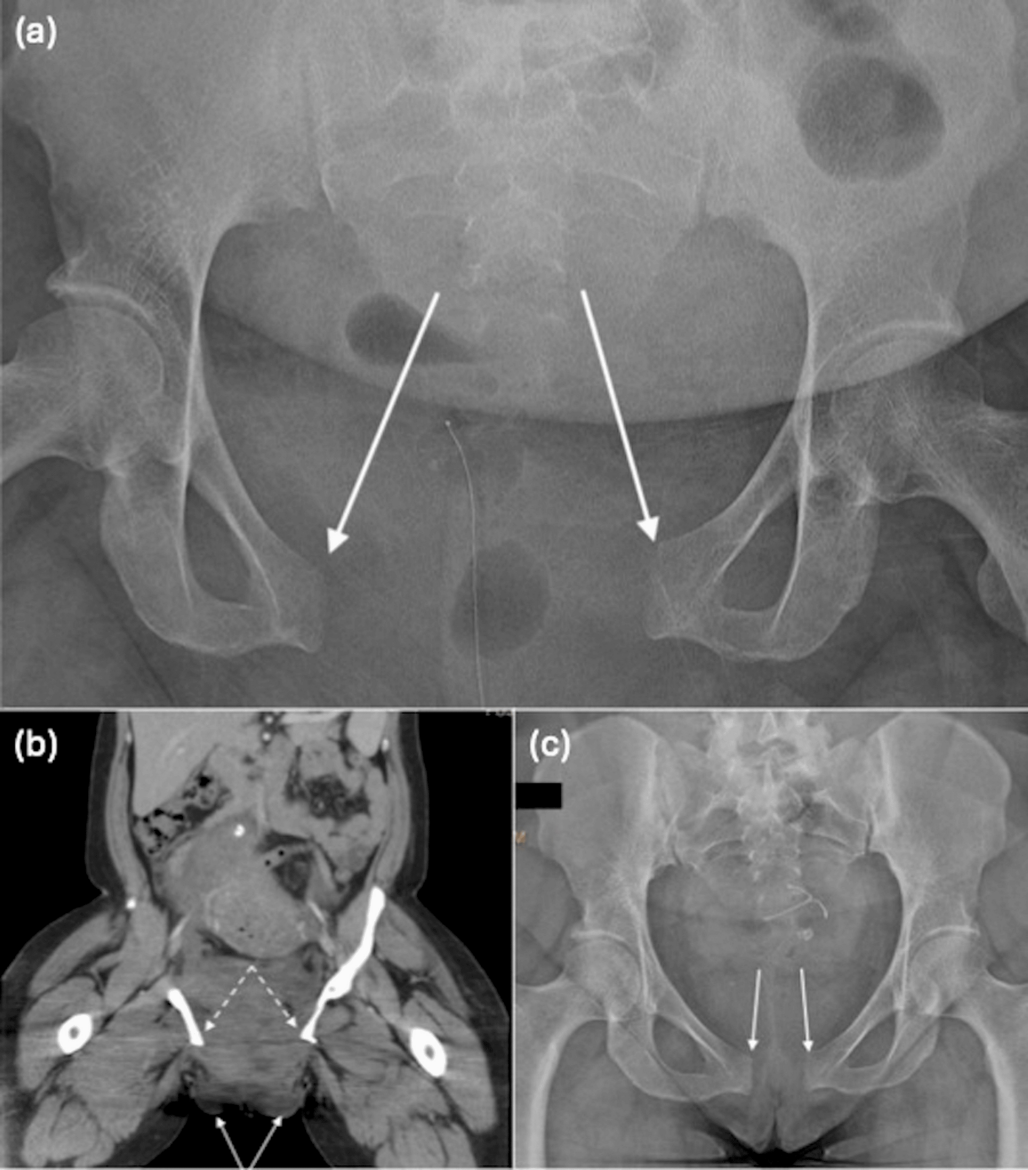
(a) Pelvic radiograph demonstrates widely separated pubic rami (arrows). (b) Computed tomography scan demonstrates labial edema and hematoma (solid arrows) at the level of pubic symphysis rupture with an 8.5 cm pubic symphysis separation (dashed arrows). (c) Pelvic radiograph after surgical management and six months following delivery demonstrates reapproximation of the pubic rami (arrows).
